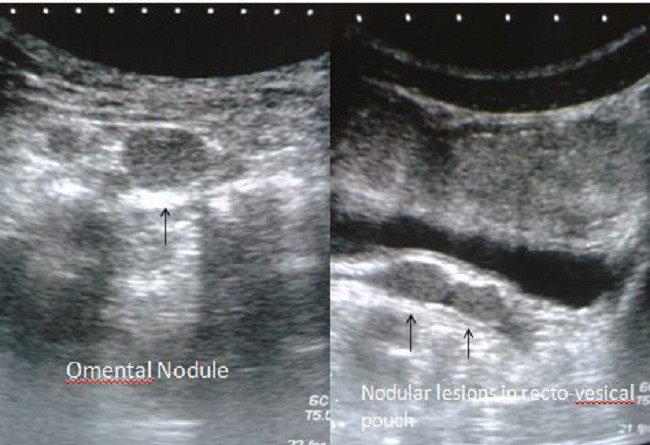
Ultrasound image in the epigastrium showing two rounded solid nodular lesions in the omentum (left) and rectovesical pouch (right)
